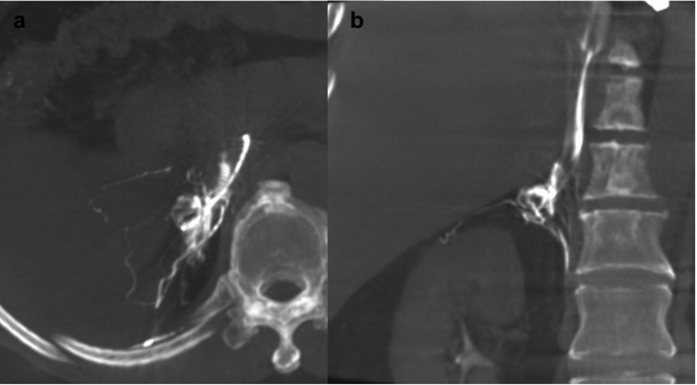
A 60-year-old man with primary aldosteronism (PA) and a right adrenal nodule. The right adrenal gland was opacified on a maximum intensity projection image on Dyna computed tomography in the transverse plane (a) and coronal plane (b). Adrenal venous sampling was successful and the lateralisation examination indicated bilateral PA.
